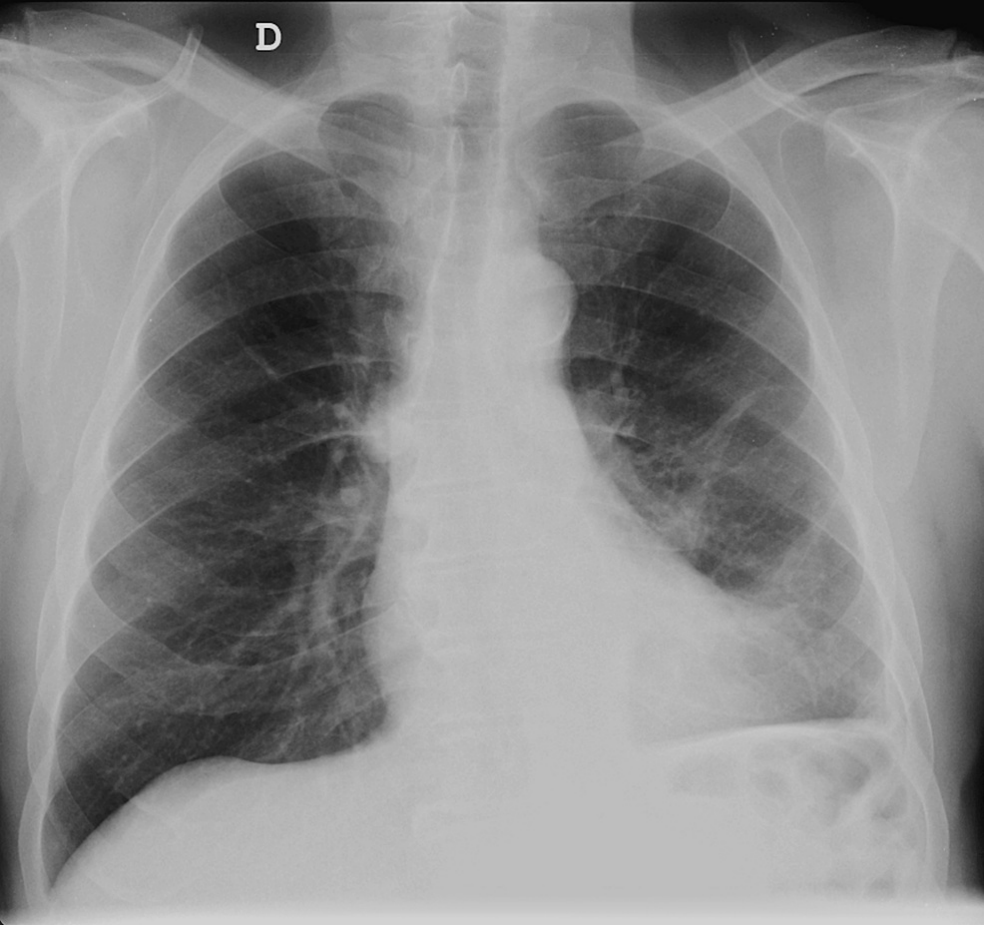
Chest X-ray three months after the episode.
